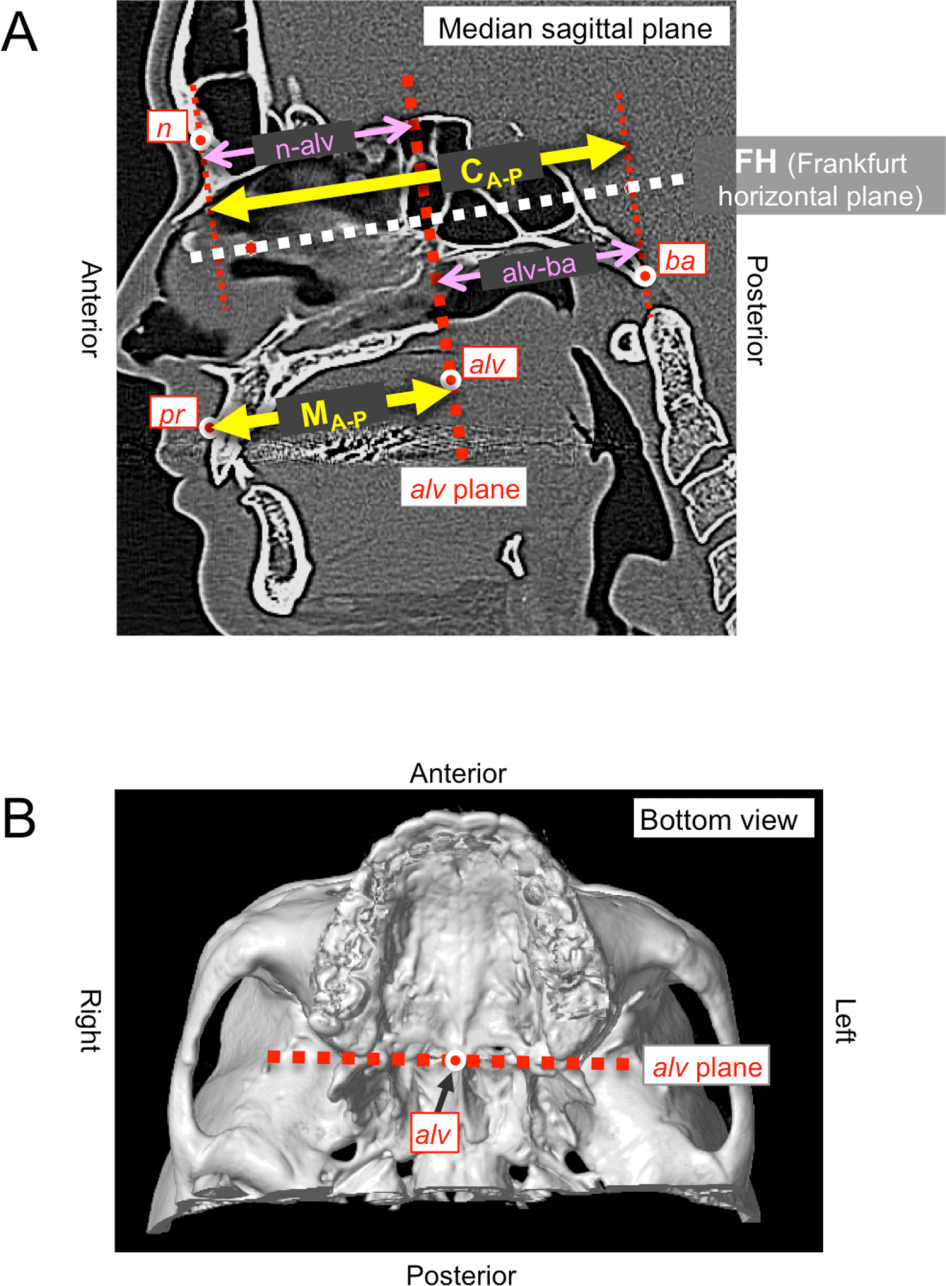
Reference points and planes, and measurement lines on the CT images. A. Measurement lines on the median sagittal plane of the cranium. The volume of interest (VOI) was limited to the maxillofacial area and the anatomical landmarks are identified. The sum of the distance from ‘*n’* to the *‘alv* plane*’* (“*n-alv* distance”) and from the *‘alv* plane*’* to *‘ba’* (“*alv-ba* distance”) was defined as a reference of the cranial anterior-posterior length, termed “C_A-P_”. The distance from *‘pr’* to the *‘alv* plane*’* was defined as the anterior-posterior length of the maxilla, termed “M_A-P_”. *‘alv’*: the point where the midline of the palate is intersected by a straight tangent connecting the posterior borders of the alveolar crests. *‘n’*: the point of intersection of the nasofrontal suture and the mid-sagittal plane. *‘pr’*: the most anterior point on the alveolar border of the maxilla. *‘ba’*: the point where the anterior margin of the foramen magnum is intersected by the mid-sagittal plane. B. Definition of ‘*alv’* plane. The coronal plane which is orthogonal to the FH (Frankfurt horizontal plane) and parallel to the line connecting the left and right *‘po’*, and passes through *‘alv’*, was defined as the *‘alv* plane*’*.
